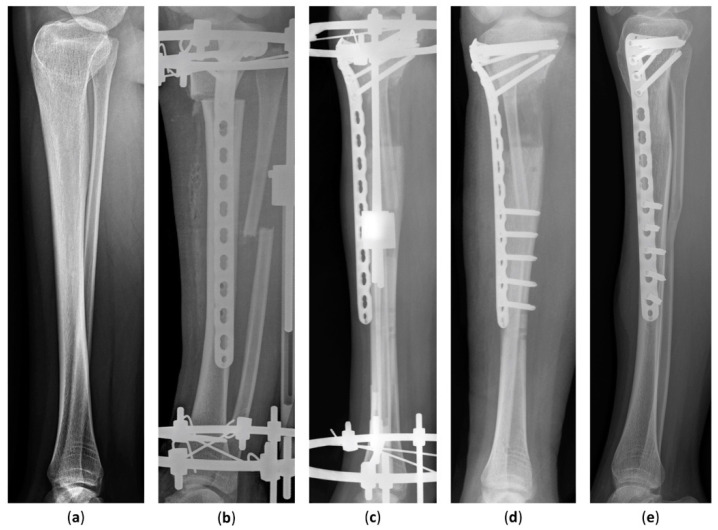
Lateral radiographs of an 18-year-old girl who underwent fixator-assisted plating and acute angular deformity correction for leg-length discrepancy. (a) A preoperative lateral view of the tibia. (b) The patient underwent tibial lengthening and deformity correction using an Ilizarov apparatus and a submuscular plate. (c) The distraction length and alignment were satisfied. (d) The distal part of the submuscular plate was fixed at removal of the external fixator. (e) Distraction osteogenesis was completed without complications 40 months after the procedure.
